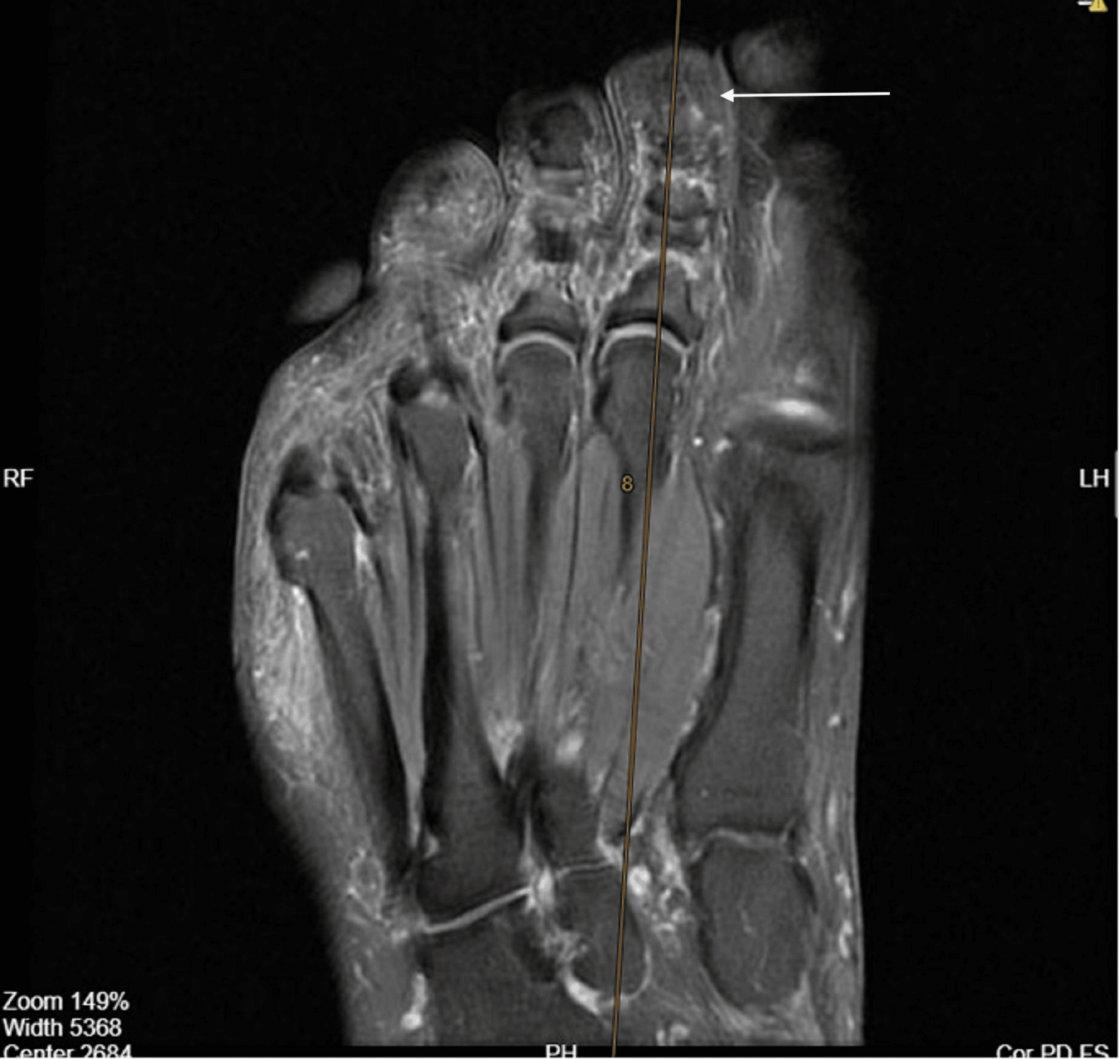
Preoperative MRI of the second toe of the right foot in coronal view (2025). There is evidence of a small, well-defined, rounded, enhancing subcutaneous nodule measuring 2.5x1.5 mm on the dorsal aspect of the tip of the second toe in the right foot, as labeled by the arrow, suggesting a vascular lesion.
